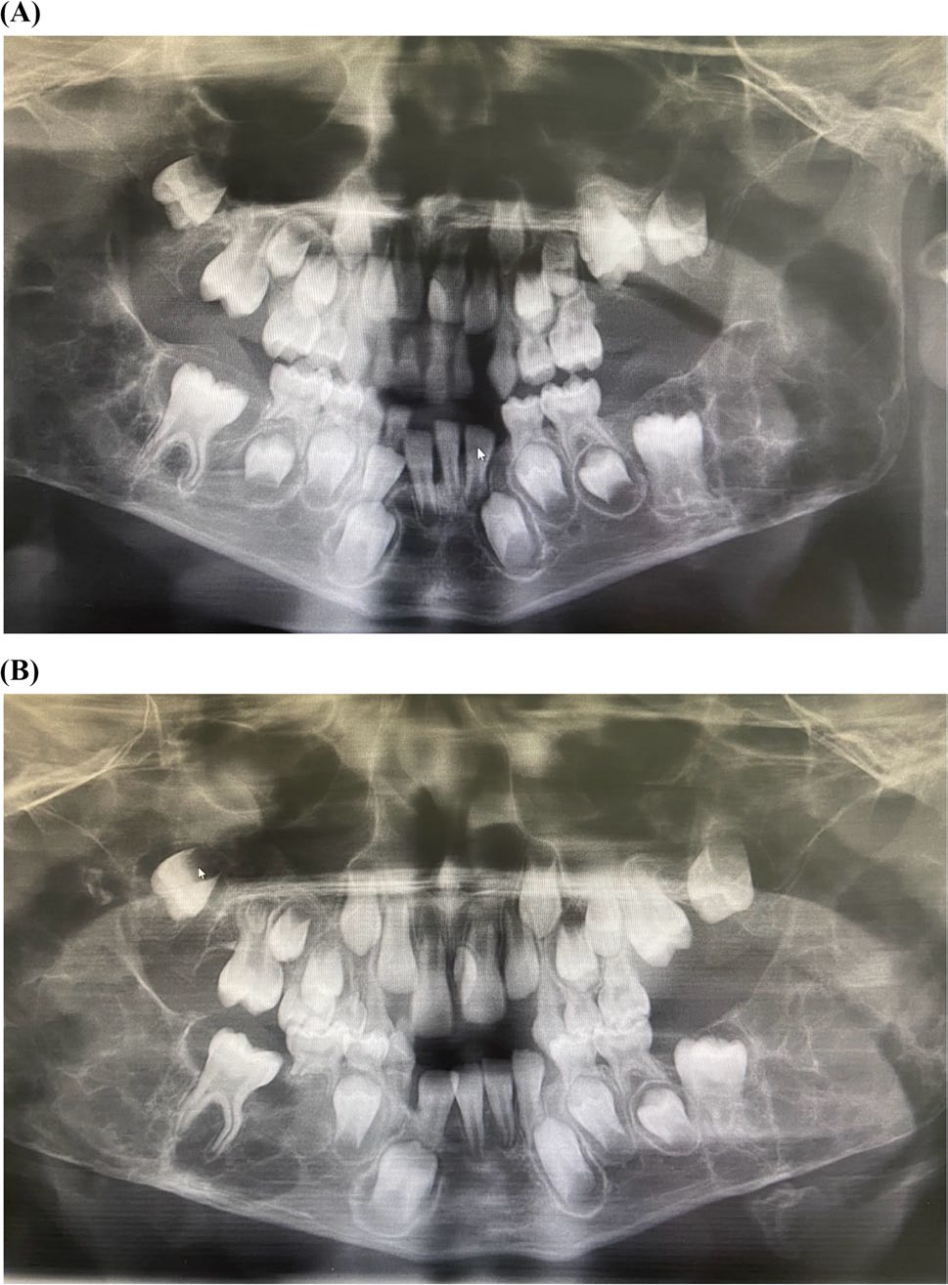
A Preoperative panoramic radiograph of bilateral, multilocular osteolytic lesions in a 7-year-old boy with NF1. B Postoperative panoramic radiograph of bilateral, multilocular osteolytic lesions in a 7-year-old boy with NF1
